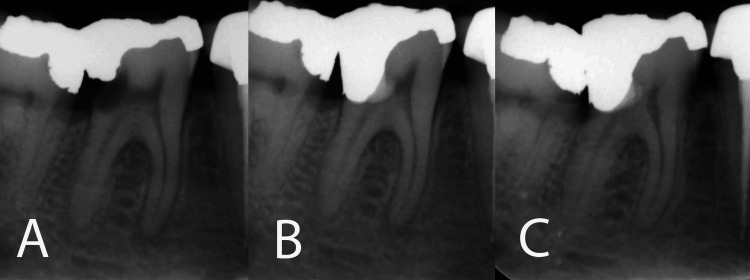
Radiographic progression of the treated case (A) Preoperative radiograph showing the involved tooth with deep caries cavity and a radiolucent apical lesion/PDL widening in the mesial/distal roots, respectively. (B) Immediate postoperative radiograph demonstrating the placement of calcium-enriched mixture cement after tampon pulpotomy. (C) One-year follow-up radiograph revealing the reestablishment of a normal PDL, indicating successful pulpal healing and resolution of apical pathology. Notably, a thick dentinal bridge is evident in front of the CEM biomaterial, separating the related pulp chamber of the mesial roots.
